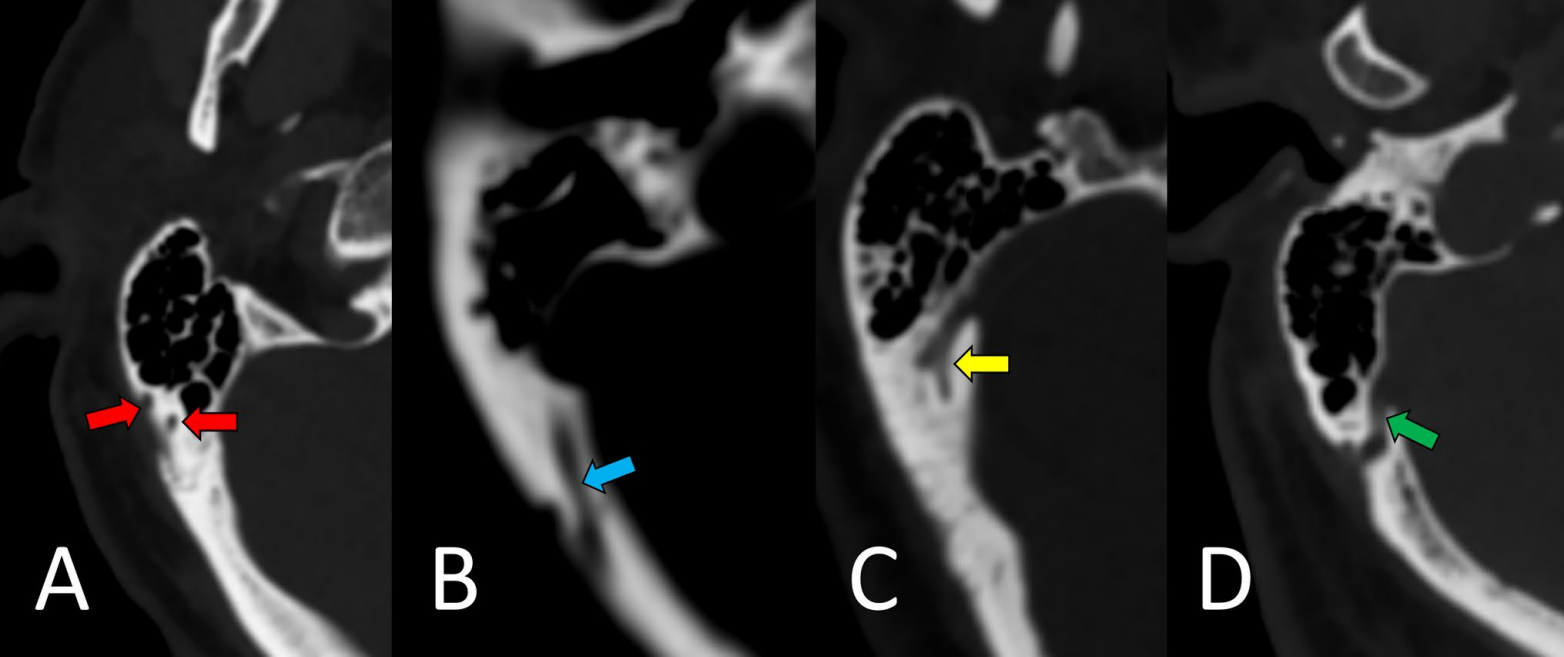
Axial slice CT images demonstrate a variety of morphologies of MECs, A two evident MEFs (red arrows), B a long MEC (blue arrow), C a single MEC that divides into two MECS with a bifurcation (yellow arrow), D and a MEC with a large diameter (green arrow), showing examples of diverse anatomical features
